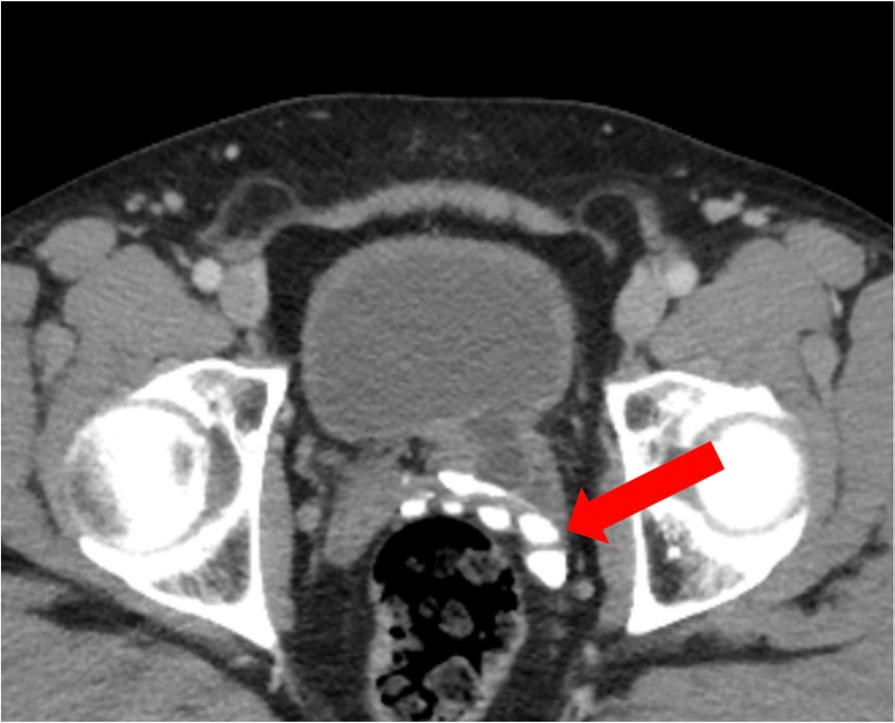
Computed tomography, transverse view demonstrating calcification of the left seminal vesicle
